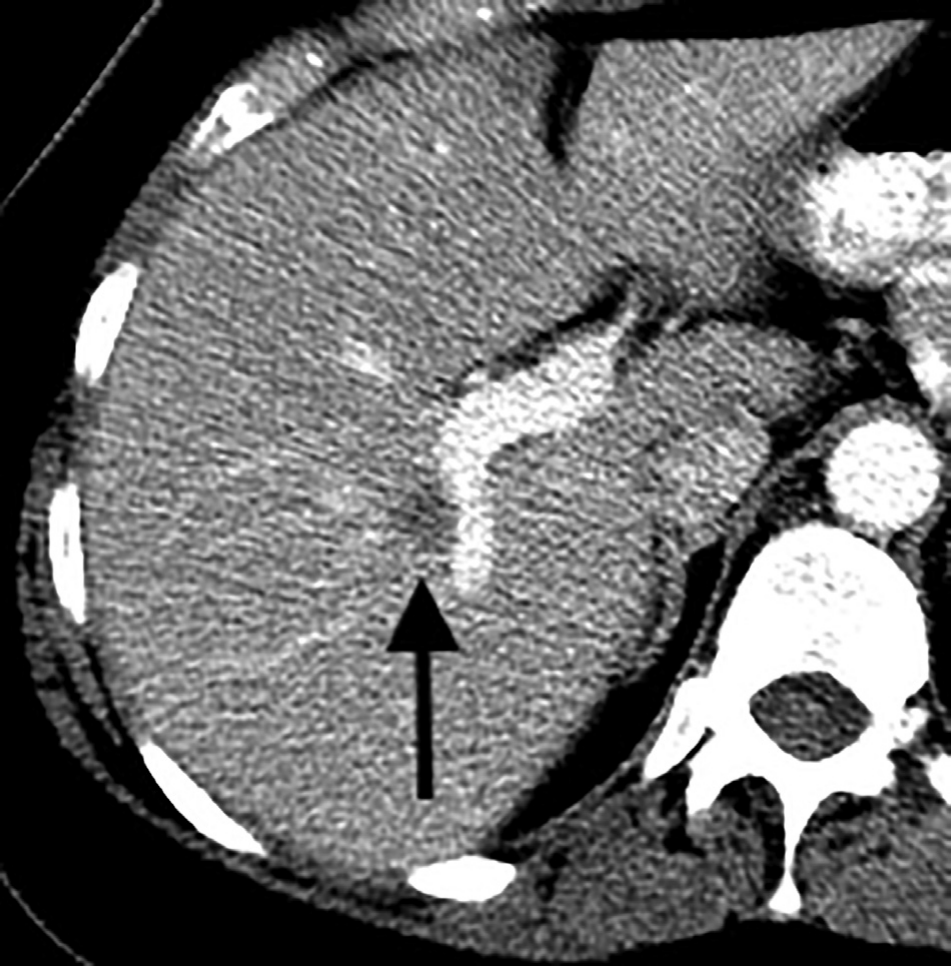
Contrast-enhanced CT demonstrating a 1.1 cm hypoattenuating lesion in the right hepatic lobe abutting a branch of the right portal vein (arrow).
